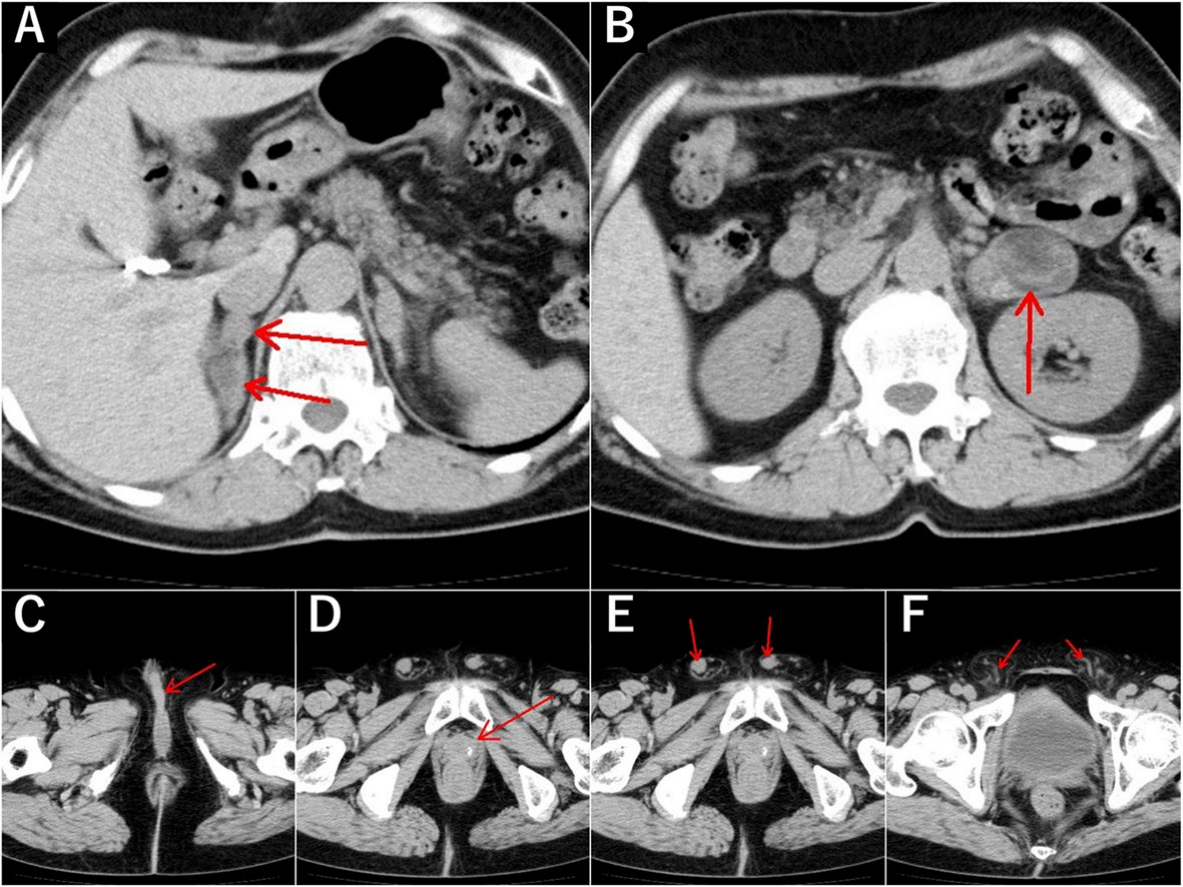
Abdominal and pelvic computed tomography scan. A, B Both adrenal glands are enlarged. C The urethral spongiosa is incompletely developed. D Prostate-like structures with calcification are present. E Testicular development is incomplete. F Testicular veins are present
